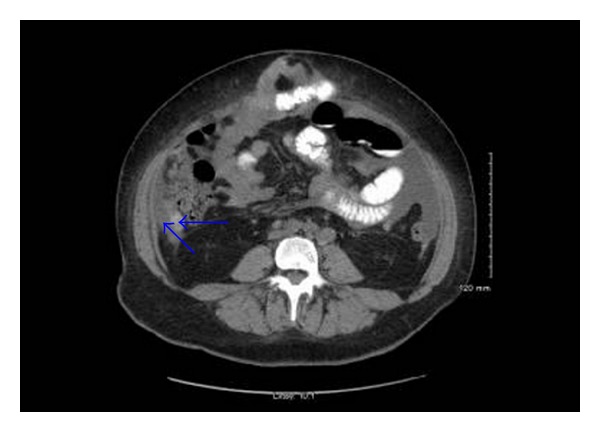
CT Abdomen without IV contrast, revealing calcified, heterogeneous, mixed intermediate, and high-density deposits worrisome for peritoneal carcinomatosis.
